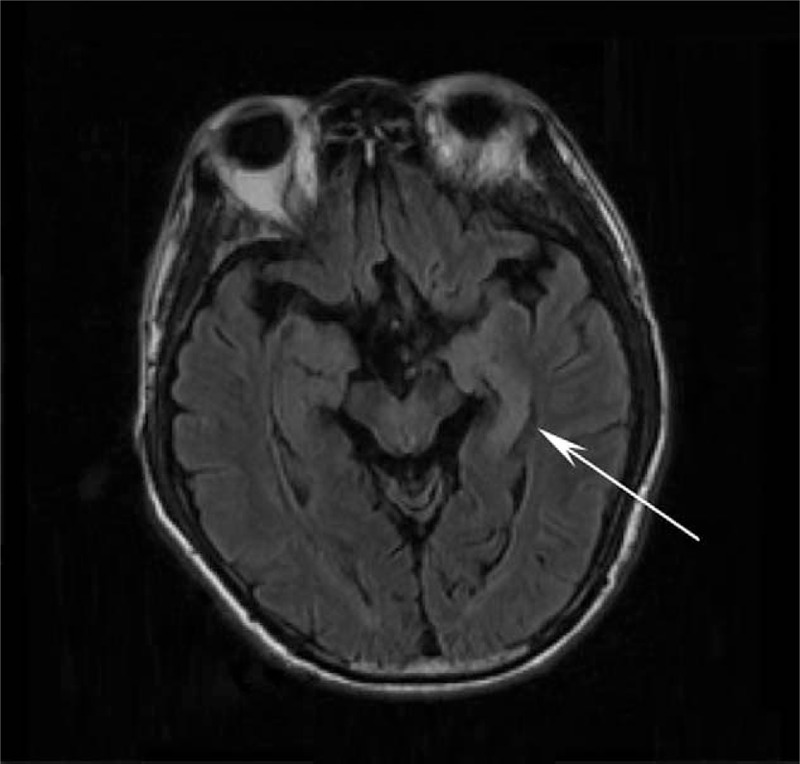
FLAIR. Cranial MRI images revealed left hyperintensity of the hippocampus on the FLAIR sequence. FLAIR = fluid-attenuated inversion recovery, MRI = magnetic resonance imaging.
